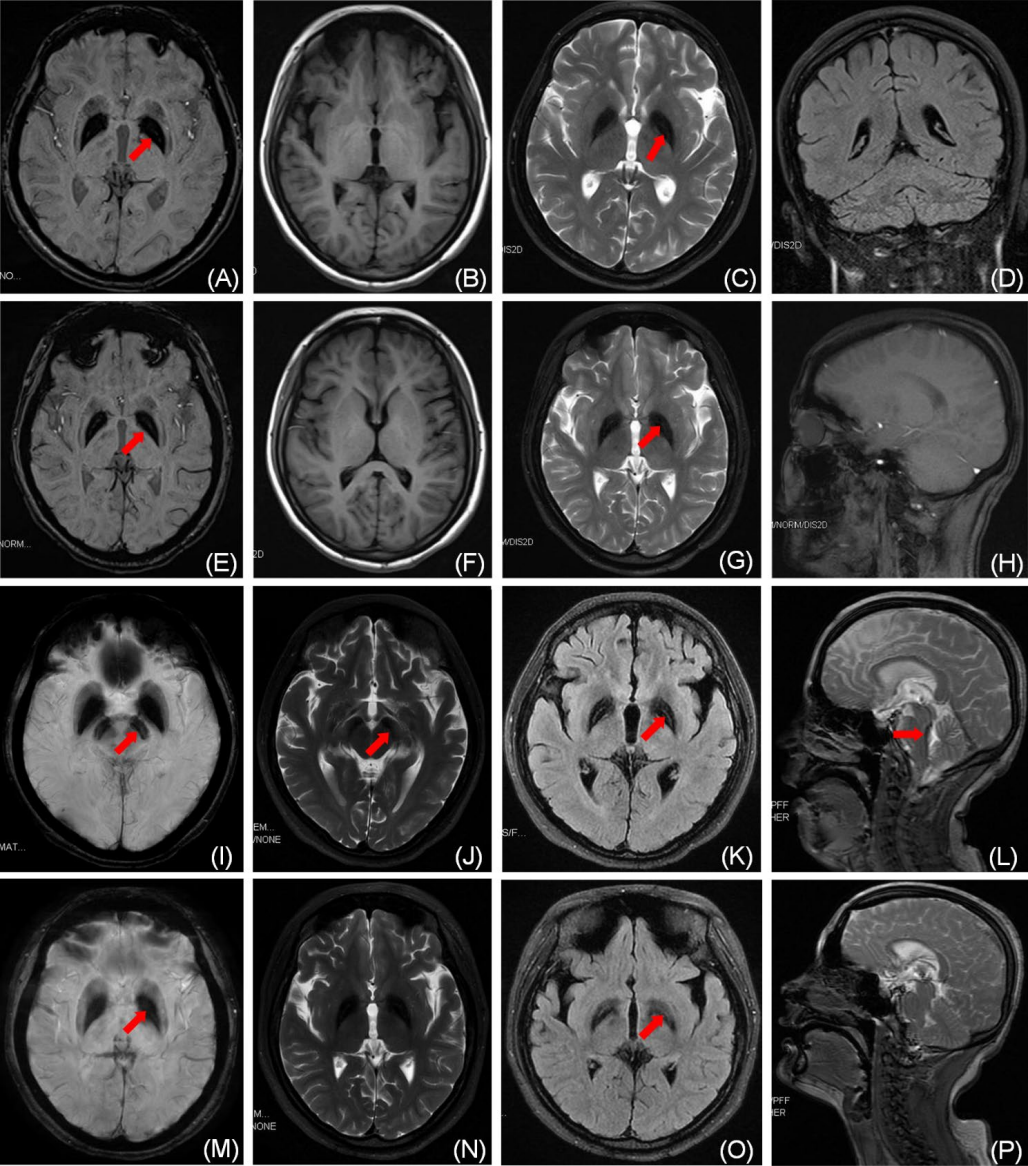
Nuclear Magnetic Resonance Imaging (A-D) show the MRI images of proband in 2014. (E-H) Shows MRI images of the proband ‘s sister at the hospital in 2014. (I-L) The MRI images of the proband in 2017; Symmetric hypodense lesions of GP and SN in T2-weighted (I) and SWI images (K) of brain imaging. (M-P) The images of her sister when she was hospitalized in 2017. The linear high signal is visible in the endomyeloid plate between the medial and lateral low signal without “tiger eye“(red arrow in figure A, C,E,G,I-L,M,O).GP, globus, pallidum; SN, substantia nigra
